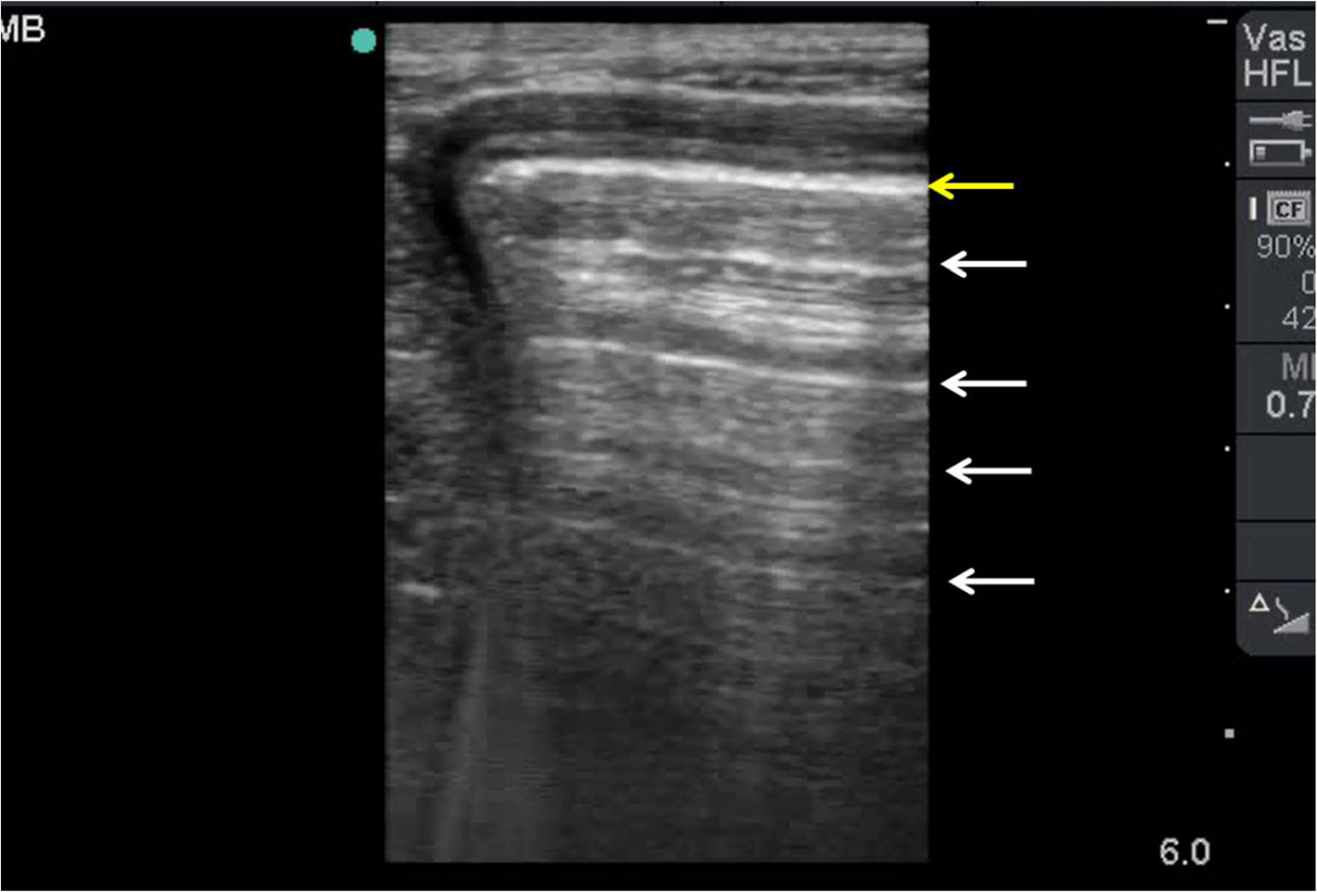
POCUS using a high-frequency linear probe for a gas-filled colon showing a reverberation artefact. This occurs when ultrasound waves bounce between the transducer and the gas. The gas is a very hyperechoic white line (yellow arrow). The reverberation lines (white arrows) represent the repetition of the reflective waves. The density of these lines becomes less as they go deeper while the distances between them are equal
